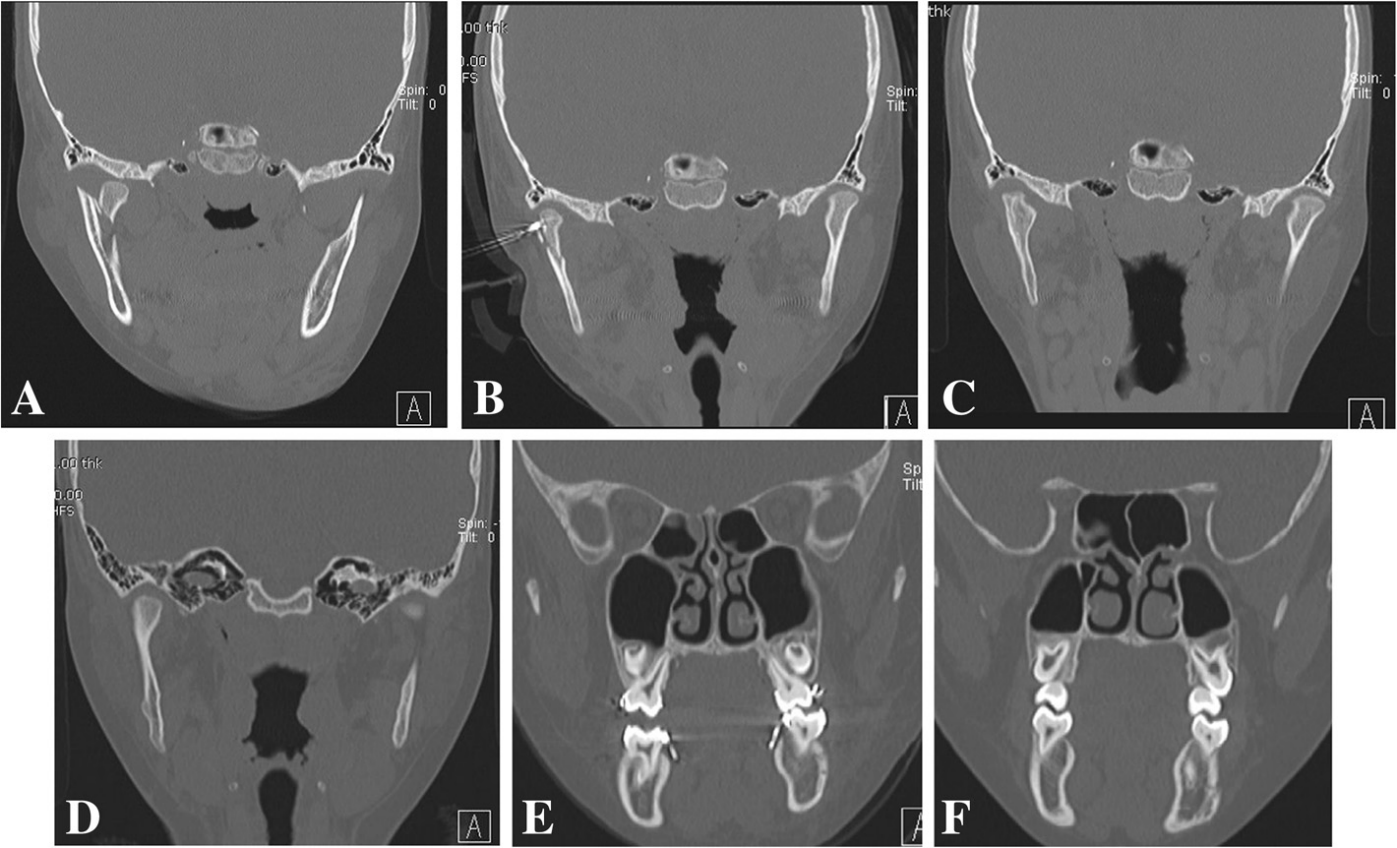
CT image of the fracture site in a pediatric patient with a condylar neck fracture. A. The fractured segment was displaced and dislocated in the medial direction and the proximal and the distal segments had no contact with each other. B. Percutaneous threaded Kirschner wires were placed in the condylar fracture segment under direct vision through the Risdon approach. The fractured segment was reduced to its original position. C. CT findings six months postoperatively. D. CT findings two years postoperatively show excellent union of the condylar neck with the normally shaped condylar head. E. CT findings preoperatively show an open bite on the injured side. F. CT findings postoperatively show normal occlusion.
